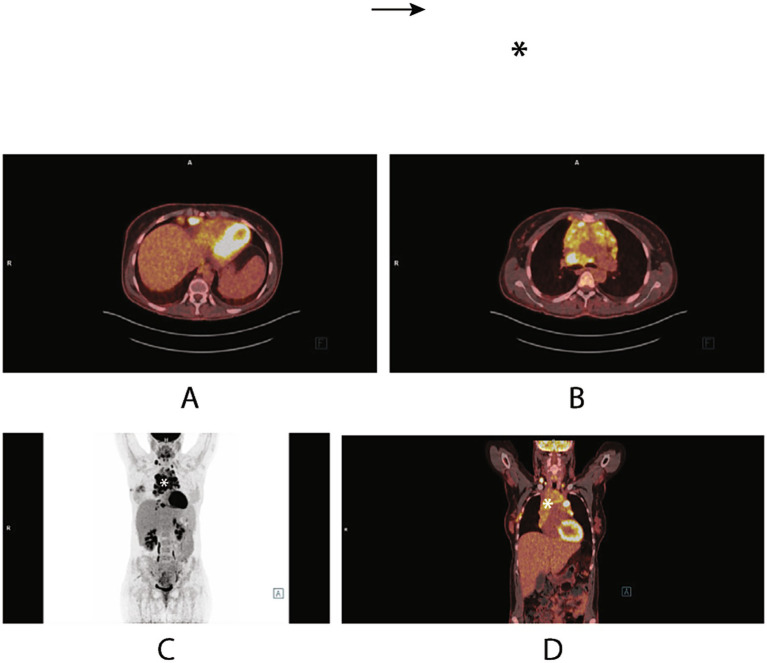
Postpartum PET/CT scan demonstrating metabolically active lymph nodes in the neck and a bulky mediastinal mass (*), consistent with lymphoproliferative disease. Additional focal uptake is seen in individual lymph nodes within the supradiaphragmatic paracardiac fat and preesophageal region. A nodule in the S3 segment of the right lung is noted but too small for characterization. Bilateral pleural effusions are present without FDG uptake. Hepatosplenomegaly is also observed.
